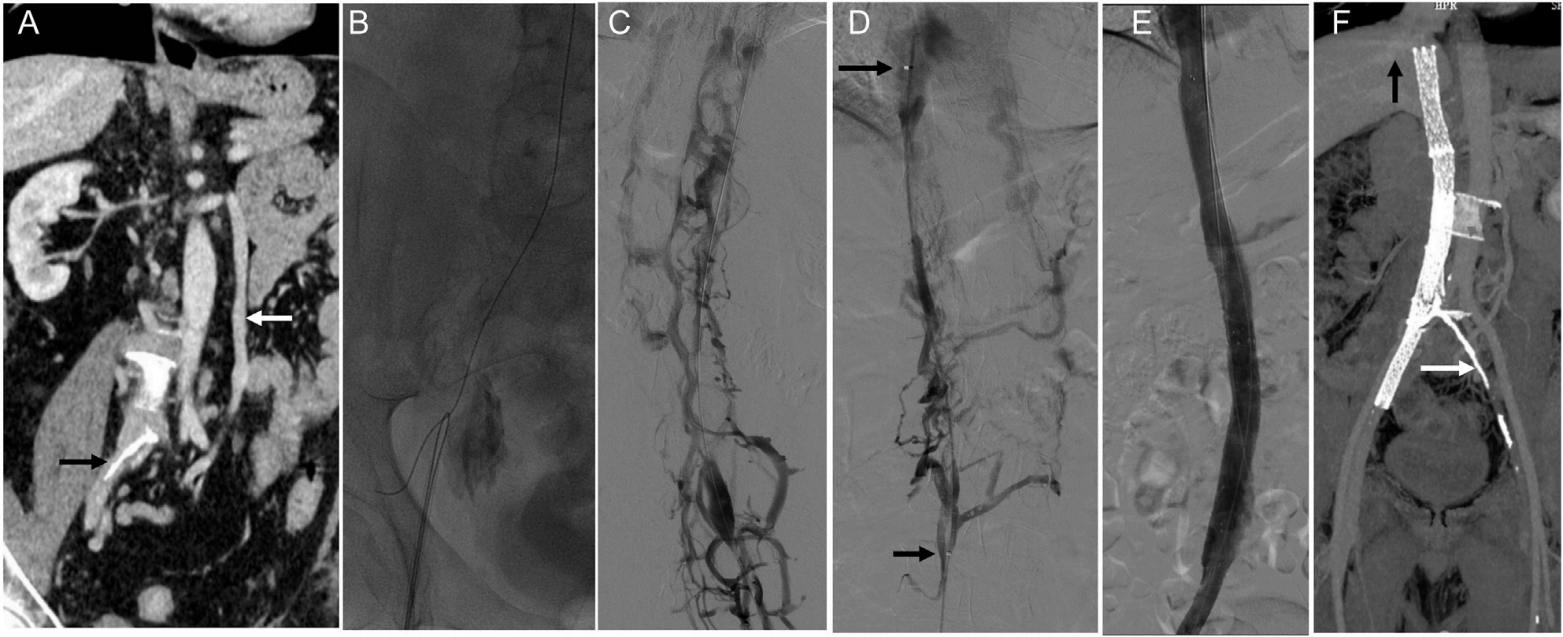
Innovative treatment and 1-year follow-up outcomes of IVC stenosis. Note: (A) A CT scan revealed a complete occlusion of the IVC, extending from the bilateral iliac veins to the entrance of the right atrium. The white arrow indicates the compensatory dilatation of the left lumbar vein, while the black arrow points out the calcified region of the iliac vein. (B) After unsuccessful attempts to access the occlusion via the femoral vein, a stiff wire and a guiding catheter were successfully inserted into the obstructed IVC through the right internal jugular vein. Subsequently, the wire was removed from the groin. (C) Femoral venography revealed the presence of abundant collateral circulation, which raised doubts about the correct placement of the wire in the IVC. (D) A thrombolysis catheter was successfully placed in the IVC, and the position of the catheter in the true lumen was confirmed through venography. The distance between the two arrows indicates the effective length of the thrombolysis catheter. (E) Gradual dilation of the IVC was performed using a balloon. Despite the dilation, the imaging results showed that the stenosis of the IVC still exceeded 50%. Therefore, four Wallstents and two Luminex stents were placed between the hepatic and right external iliac veins. After the stents were placed, subsequent imaging results demonstrated the restoration of patency in the IVC. (F) The one-year follow-up CT scan confirmed the IVC and right iliac vein patency with no residual stenosis. The black arrow in the image points to the hepatic vein, while the white arrow indicates the calcified region of the iliac vein.
